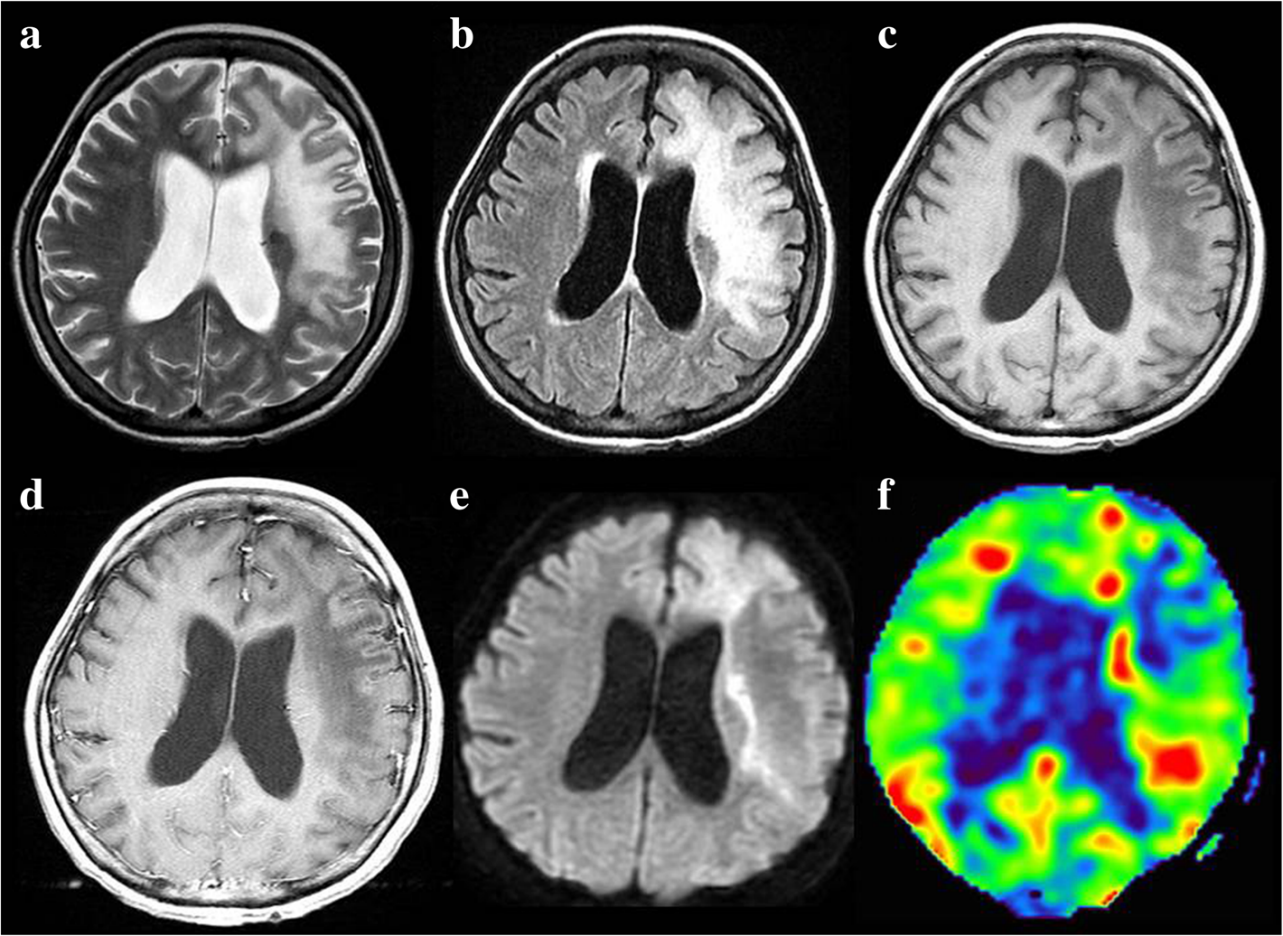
Brain magnetic resonance imaging on admission (a-e) and at 30 days after admission (f). T2-weighted images (a) and fluid-attenuated inversion recovery images (b) show hyperintense lesions in the left frontal white matter. These lesions were hypointense on T1-weighted images (c). There was no enhancement on gadolinium-enhanced T1-weighted images (d). Diffusion-weighted images (DWI) demonstrated a hyperintense lesion in the margin of the left frontal white matter (e). Arterial spin labeling revealed hyperperfusion in the DWI hyperintense lesion (f)
